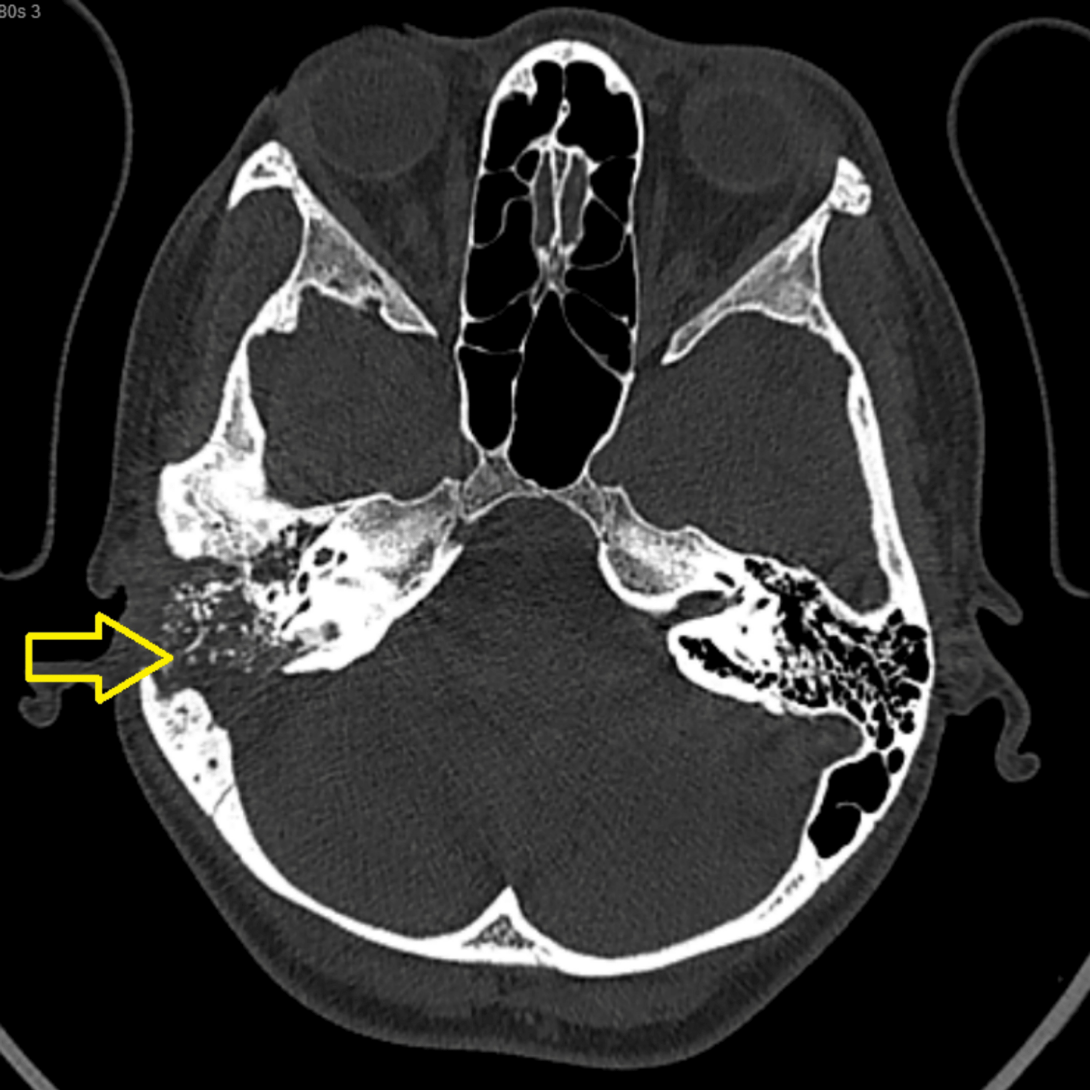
Repeated contrast-enhanced CT brain showed resolved right-sided subperiosteal mastoid collection six months post-anti-TB medication
